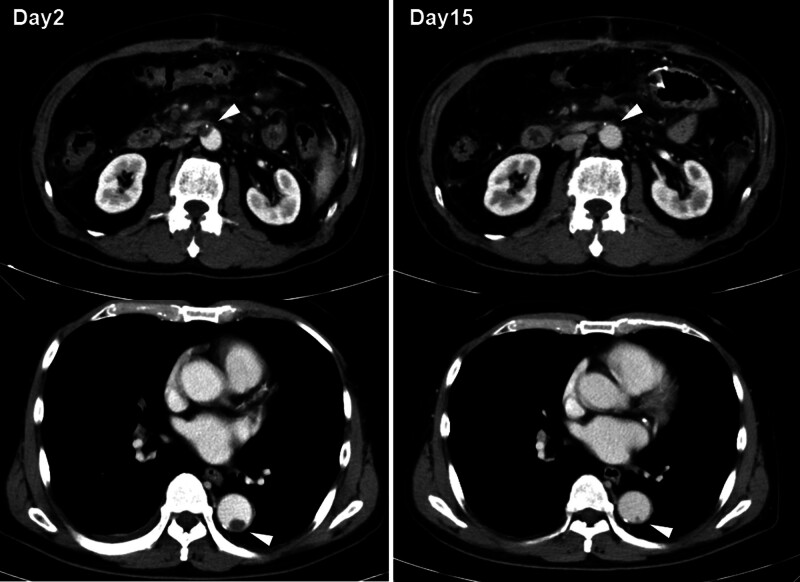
Changes in aortic thrombus before and after treatment using contrast-enhanced CT. The images show contrast-enhanced CT findings on Day 2 and Day 15. Arrowheads show the aortic mural thrombus. CT = computed tomography.
